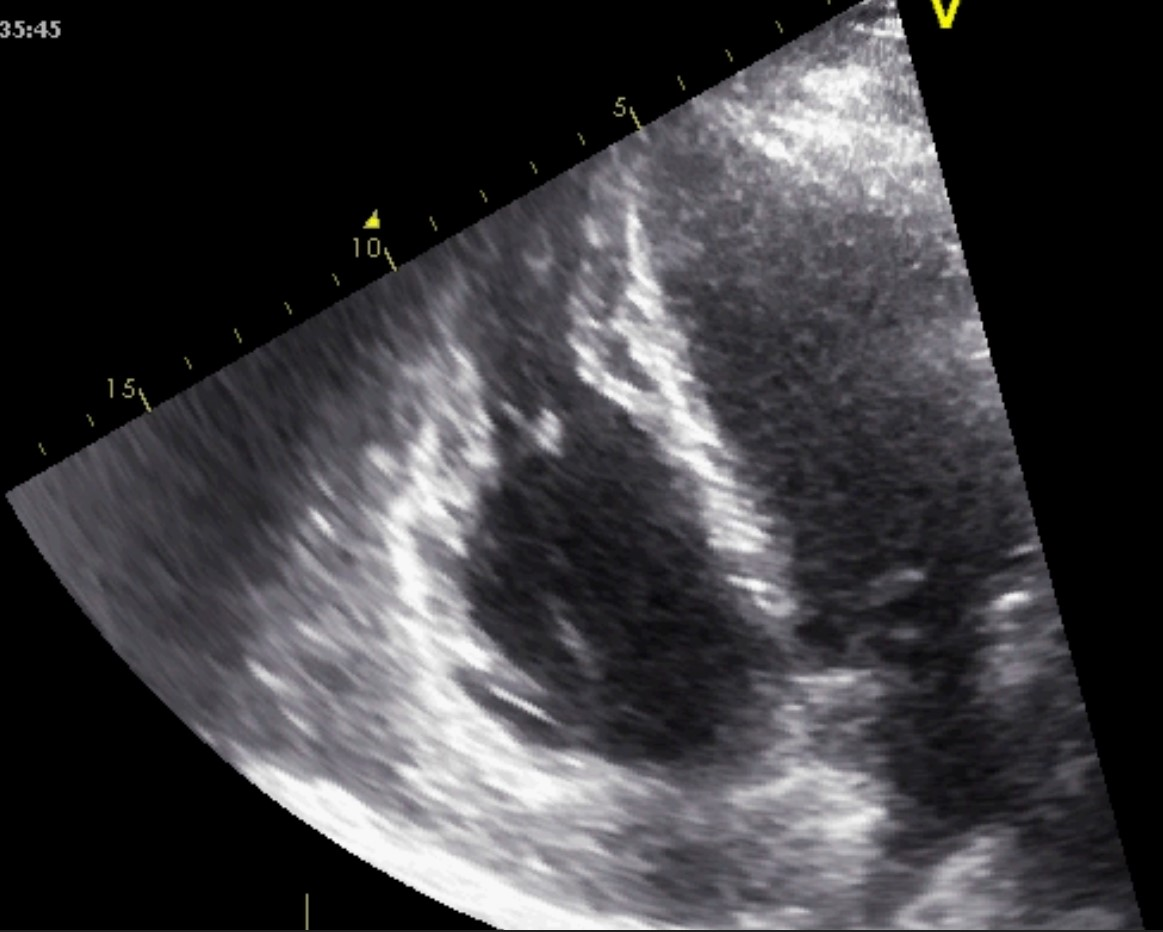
Echocardiographic aspect of AC. Apical 4 chamber view showing
